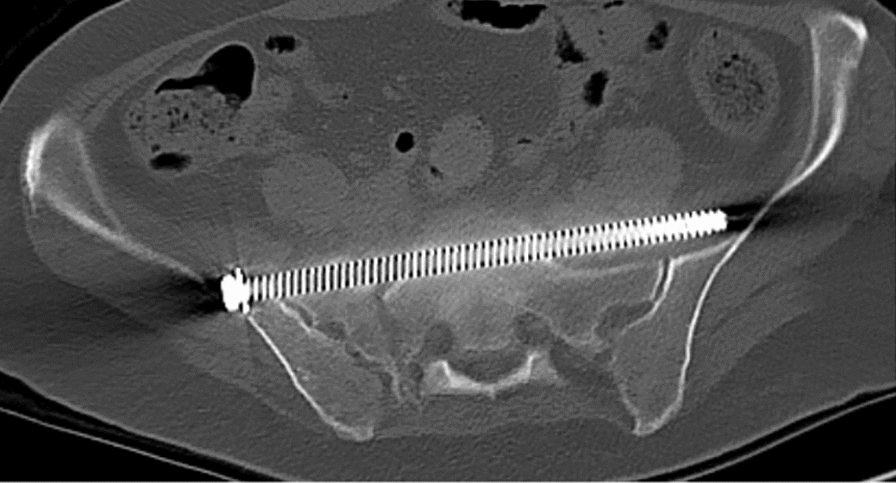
CT scan of the patients’ pelvic bones showing the misplaced screw
